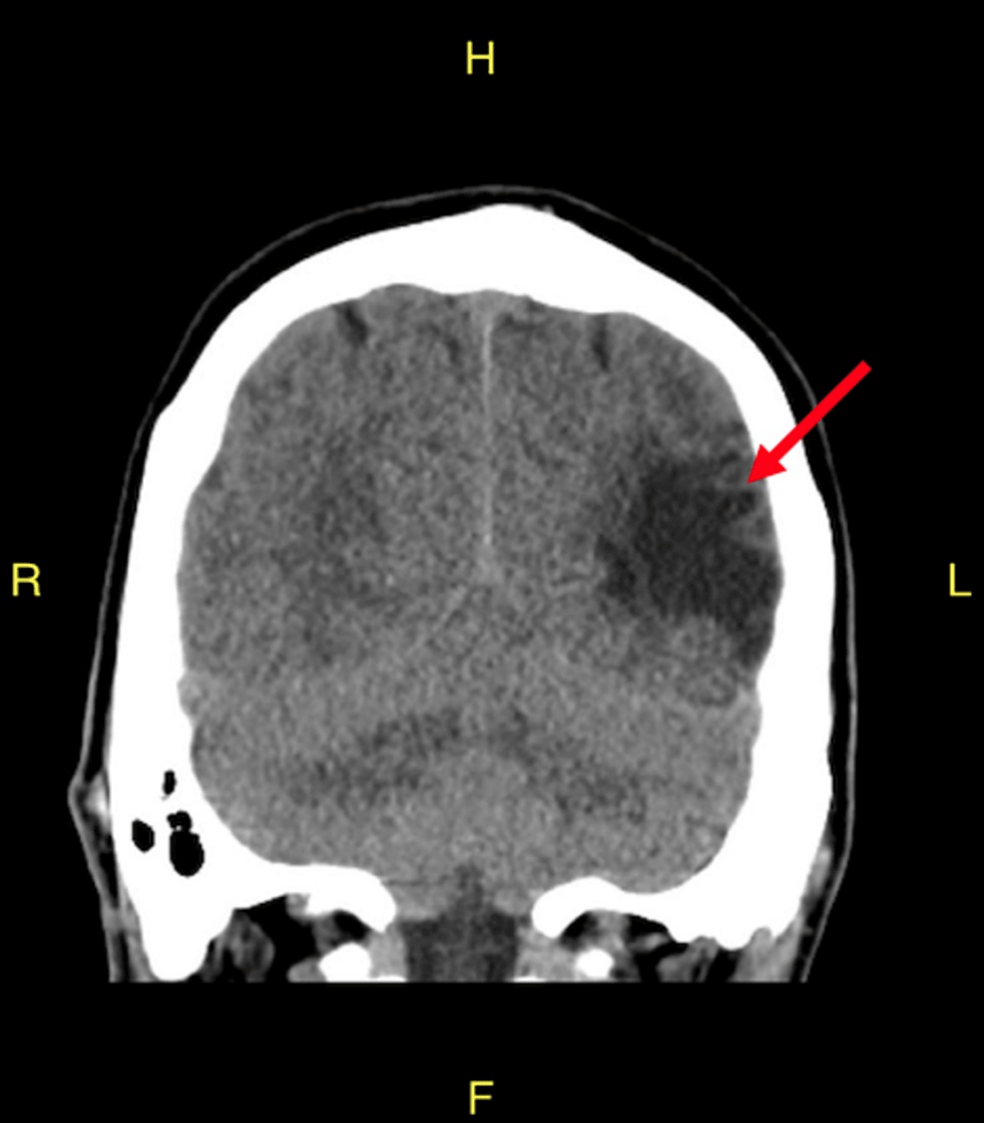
CT head without contrast (coronal view); re-demonstration of encephalomalacia in the left temporoparietal lobe from prior infarct
